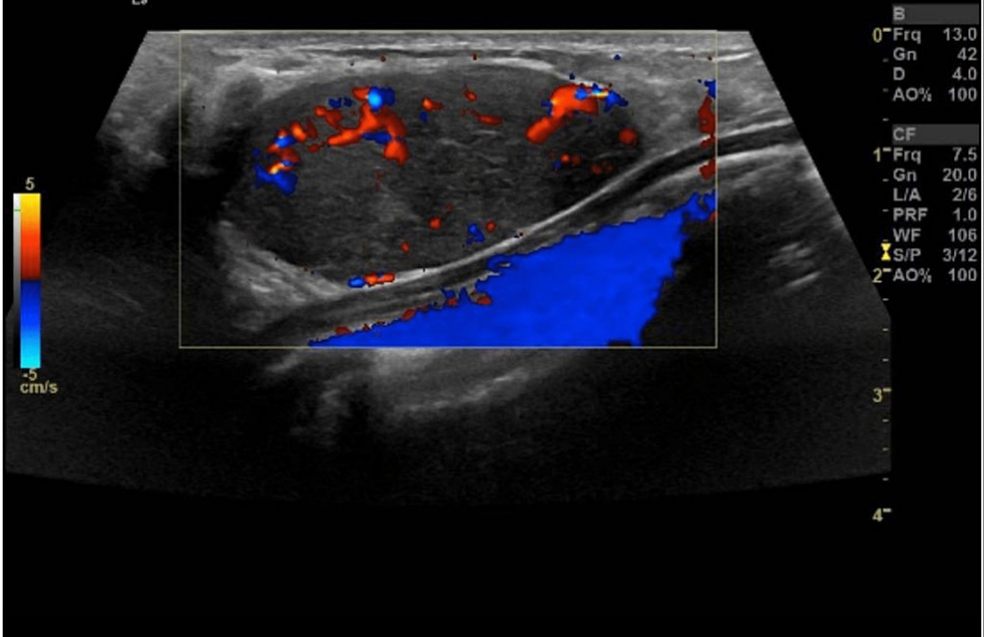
Doppler ultrasonography image of the left breast hypoechoic mass
